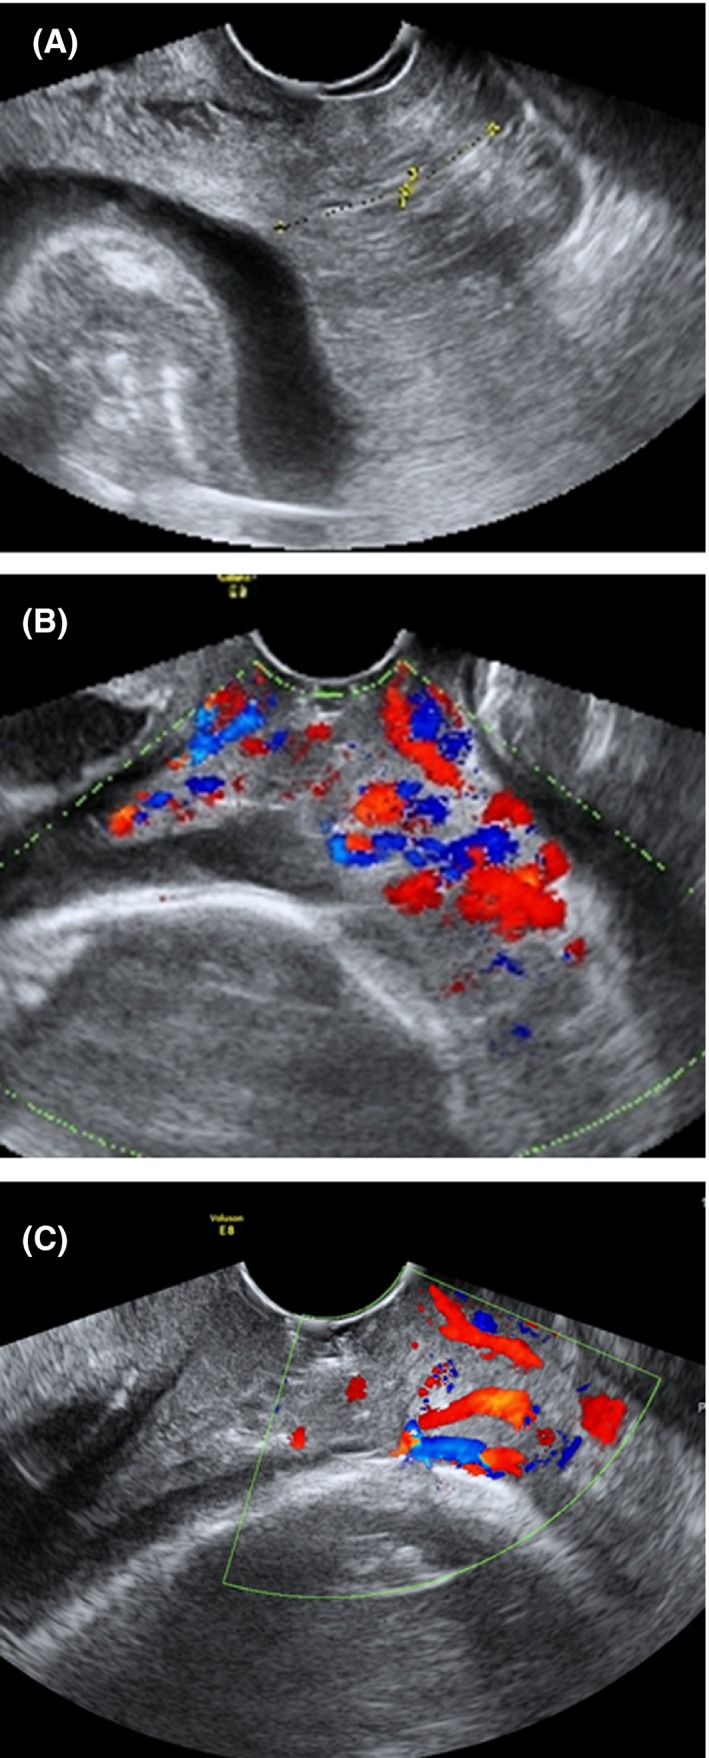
Serial measurements of the length of the ‘neo‐cervix’ were performed, and revealed that it had shortened during pregnancy. A, neo‐cervical length was 27 mm at 15^+1^ wk on admission. B, neo‐cervical length was 20 mm at 25^+6^ wk, and transvaginal ultrasonography showed abundant vascularity (red and blue) in the “neo‐cervix” region. C, neo‐cervical length was 8 mm at 31^+5^ wk and transvaginal ultrasonography showed abundant vascularity in the “neo‐cervix” region
